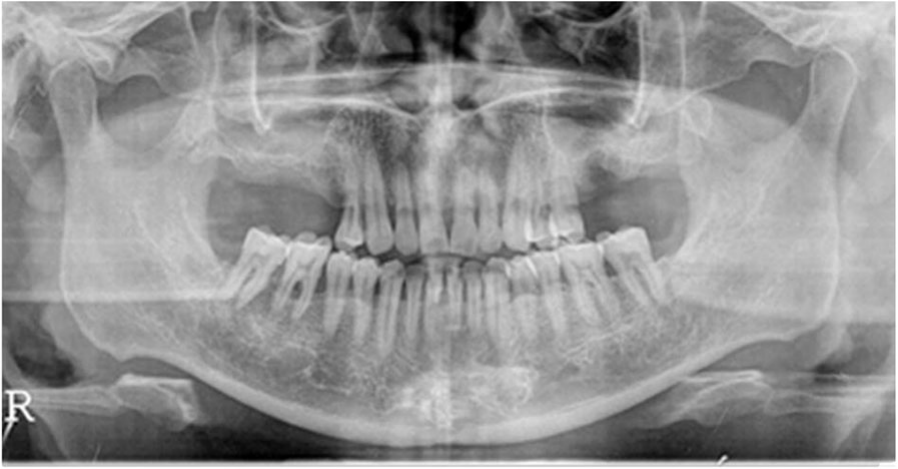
Preoperative panoramic radiograph of 46-year male patient. Residual bone height is about 2–3 mm, and sinus radiopacity is observed in the right maxilla
